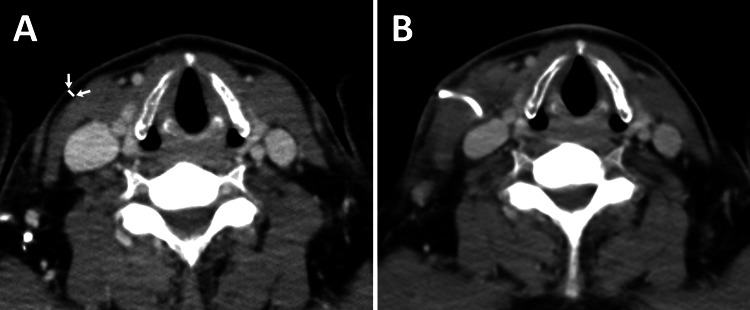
Cervical subcutaneous fat tissue thickness before central venous port implantation on CT A: Cervical subcutaneous fat tissue thickness of the subcutaneous catheter route was retrospectively evaluated on CT performed within one month pre-CVP implantation (white line between arrows). B: CT after CVP implantation.
